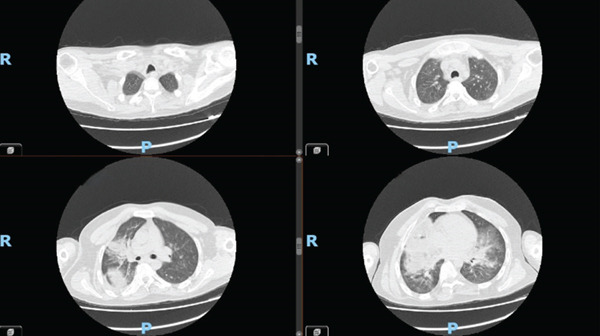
CT scan performed at discharge from ICU.
